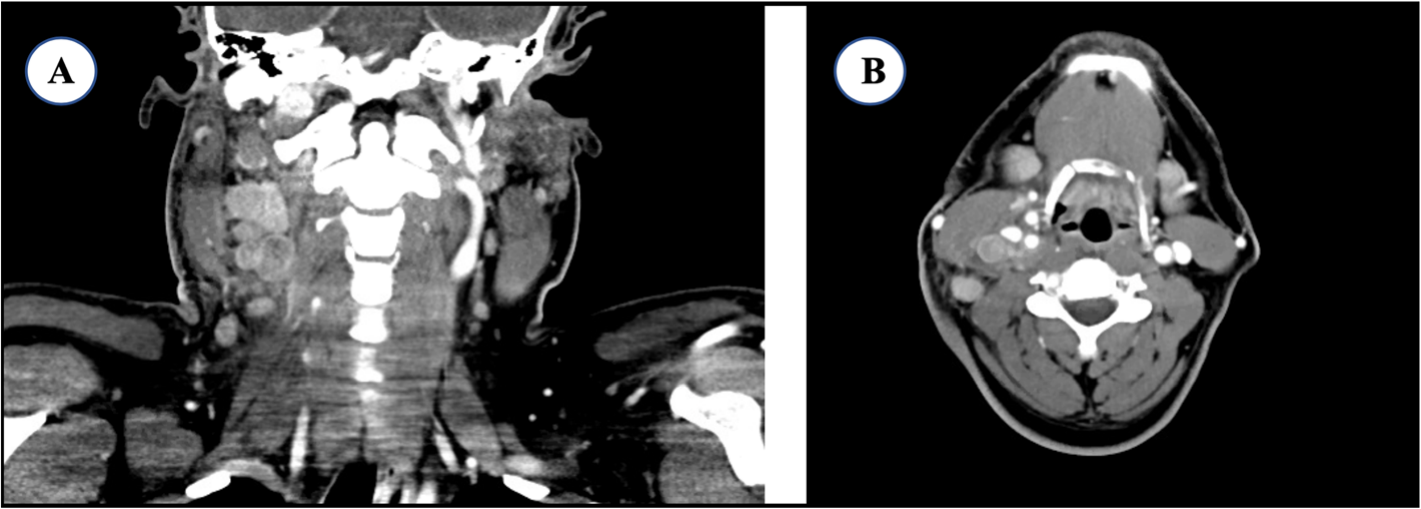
Representative coronal (a) and axial (b) cuts of a CT neck with contrast displaying right sided cervical lymphadenopathy throughout levels 2-4 along the jugular chain of lymph nodes
